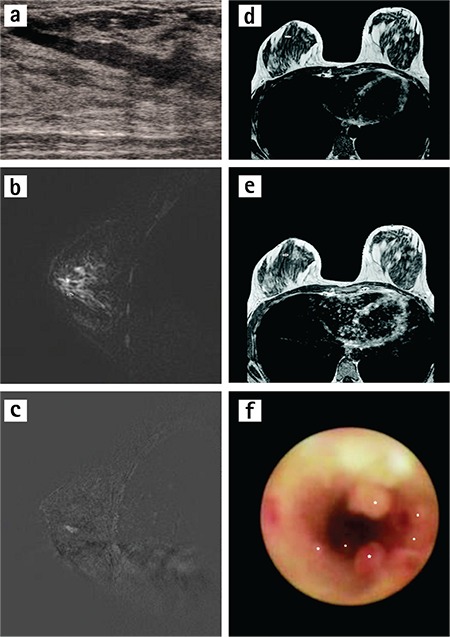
a-f. A woman aged 38 years who had had bloody discharge three times from her right breast. Pathology showed intraductal papillomatosis. Ultrasound image shows (a) shows hypoechoic masses indistinguishable from the intensive content extending along the duct. Sagittal heavily T2-weighted image (b) image shows filling defect (arrow) in distal to the duct. Sagittal T1-weighted subtraction (c) image shows non-mass linear contrast enhancement during the segment of 12 mm trace to duct. Axial T1-weighted precontrast (d) and post-contrast (e) images show contrast enhancement (arrows). After irrigation and washing; mammary ductoscopy (f) image shows papillomatosis (asterix) in the duct.
